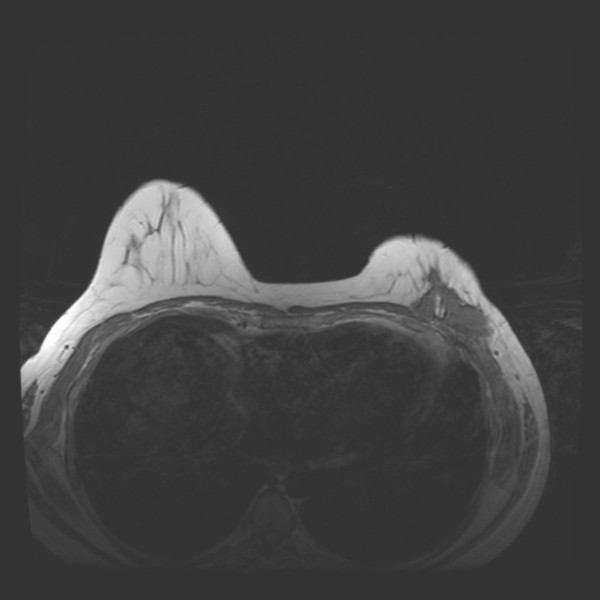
Magnetic resonance imaging non-contrast enhanced T-1 axial view.
